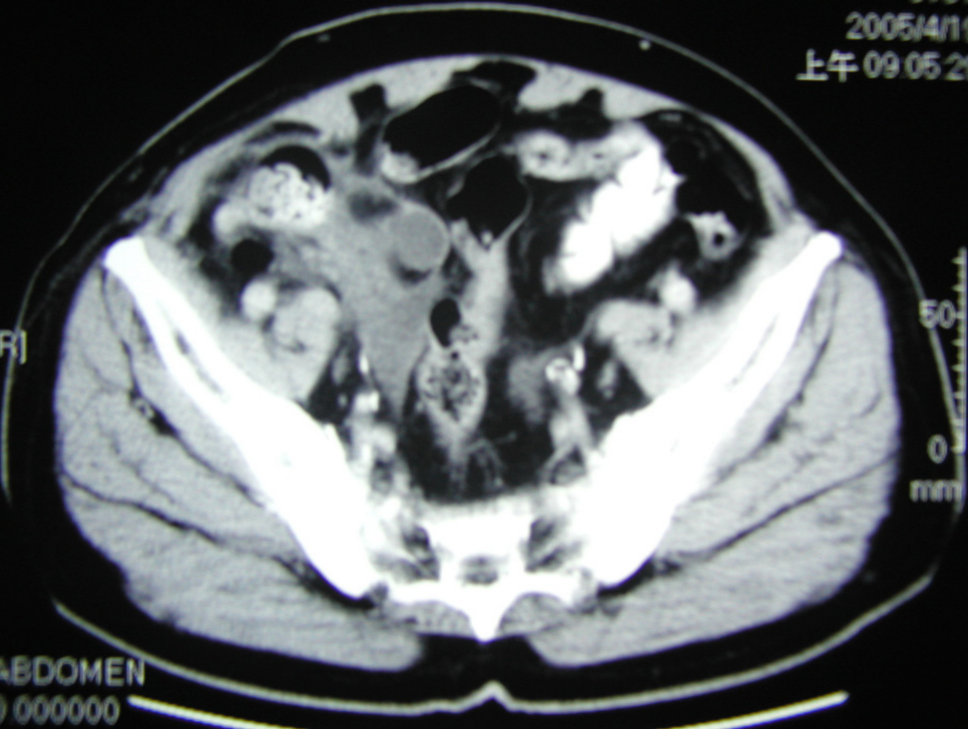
No oral contrast media leaking from the cecum into the peritoneal cavity was noted on the CT scan.
